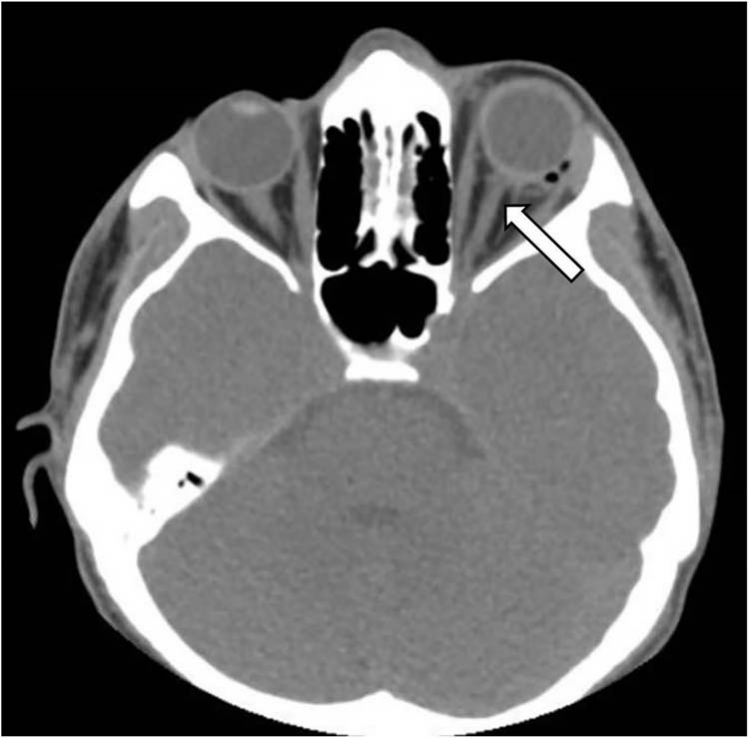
Axial section of CT-Head showing intact left optic nerve (white arrow).
